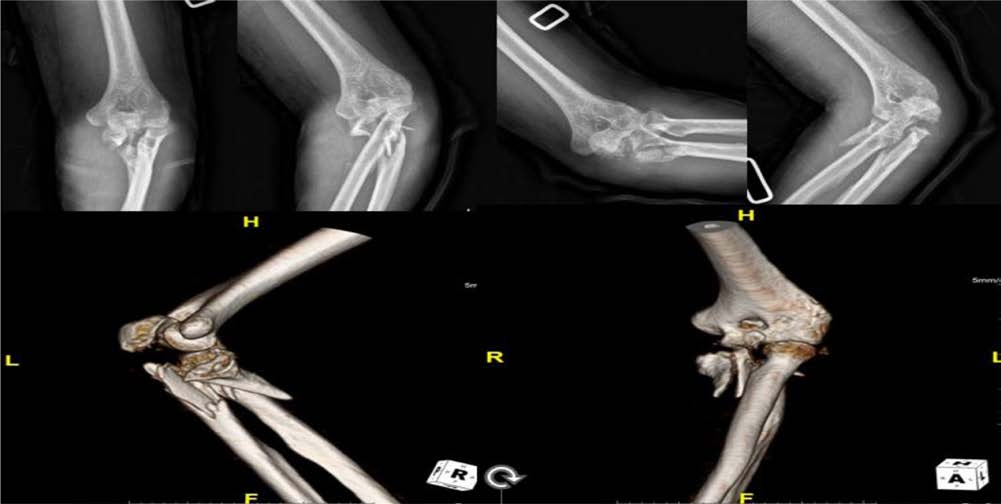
Preoperative X-ray and computed tomography showing comminuted fracture of proximal ulna including olecranon process and coronoid process.
